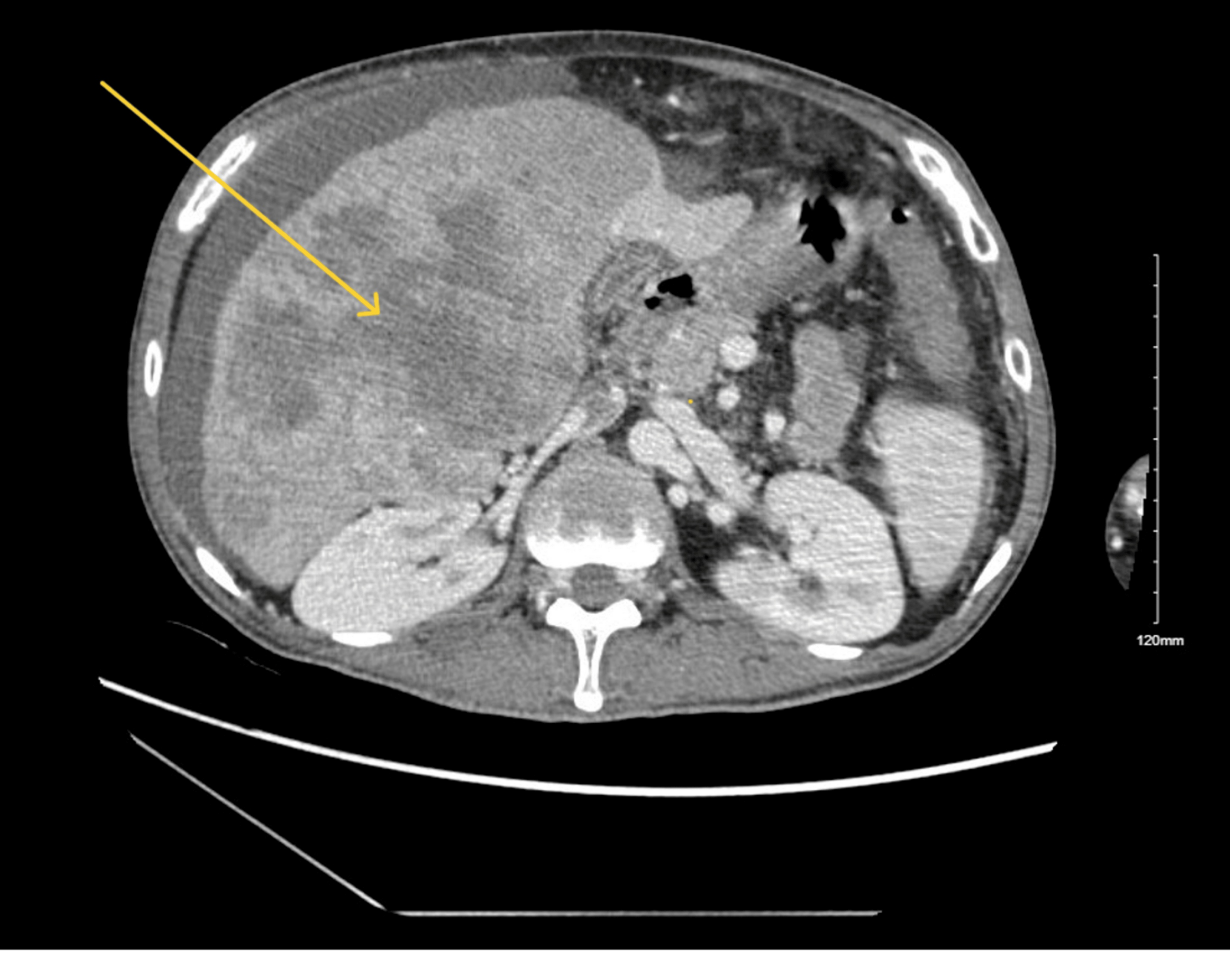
Computed tomography (CT) scan of the abdomen, transverse view showing enlarged liver with liver lesions (yellow arrow)
